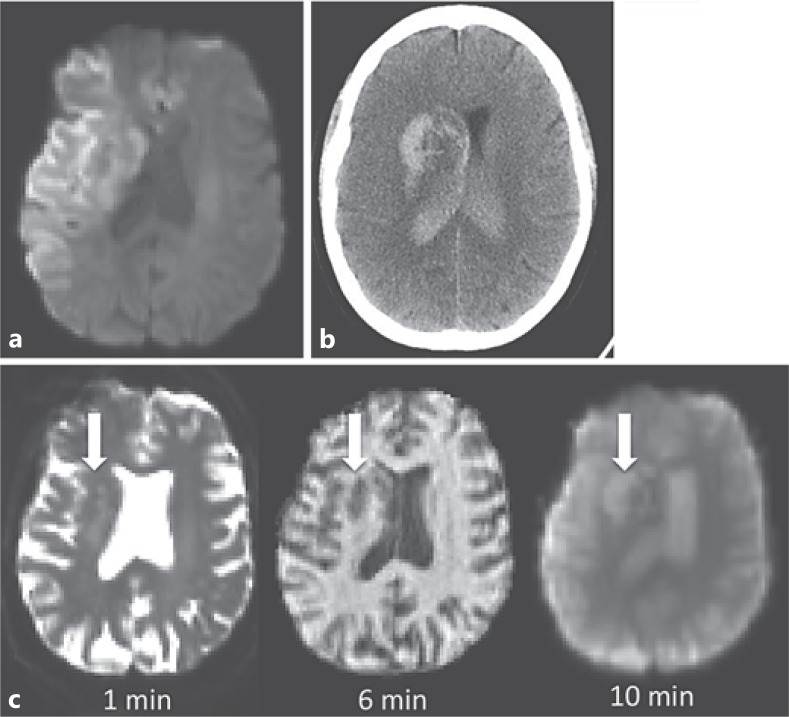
a Diffusion-weighted imaging 95 min after symptom onset demonstrating a large volume of tissue infarction within the right hemisphere. b Noncontrast CT scan at 180 min showing hemorrhage within the right basal ganglia and extension into both lateral ventricles. c Serial coregistered images during the MRI showing the appearance and growth of hematoma (arrows) over time from the beginning of the scan. Diffusion-weighted sequence: b = 0 s/mm^2^; T1-weighted image; arterial spin-labeled perfusion image, unprocessed data.
